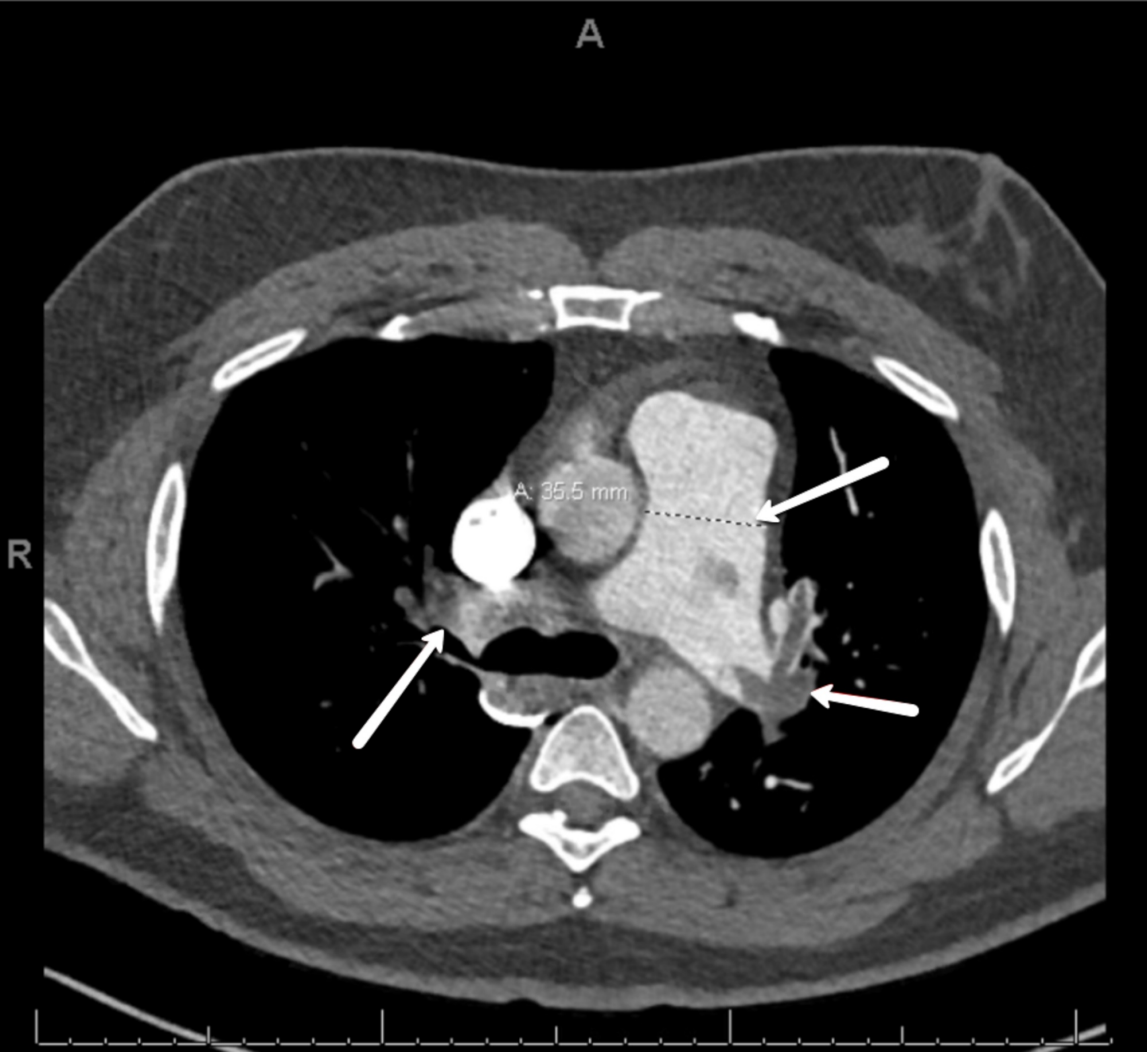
CT. Obstruction of right and left pulmonary arteries by thrombi. Dilation of the pulmonary artery to 35.5 mm.
